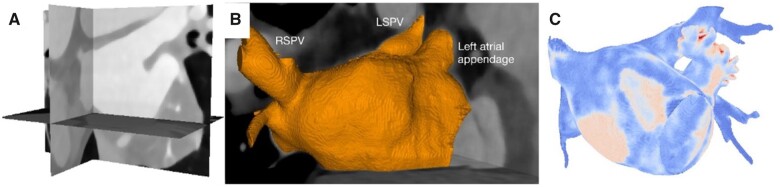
Multiple advantages of pre-procedural contrast-enhanced computed tomography imaging to inform atrial fibrillation ablation. (*A*) Contrast-enhanced computed tomography imaging, which is routinely and widely performed to exclude left atrial appendage thrombus prior to left atrial ablation. (*B*) 3D segmentation of left atrial blood pool, which may be rapidly acquired from contrast-enhanced computed tomography imaging using open-source software or proprietary programs within the commercially available electroanatomic mapping systems. Computed tomography-derived left atrial anatomy provides structural information about left atrial appendage size, shape and the configuration of pulmonary veins which may be helpful during an atrial fibrillation ablation procedure. (*C*) Experimental use of contrast-enhanced computed tomography imaging to calculate a global left atrial wall thickness map. Computed tomography-derived tissue thickness may provide additional substrate information.
